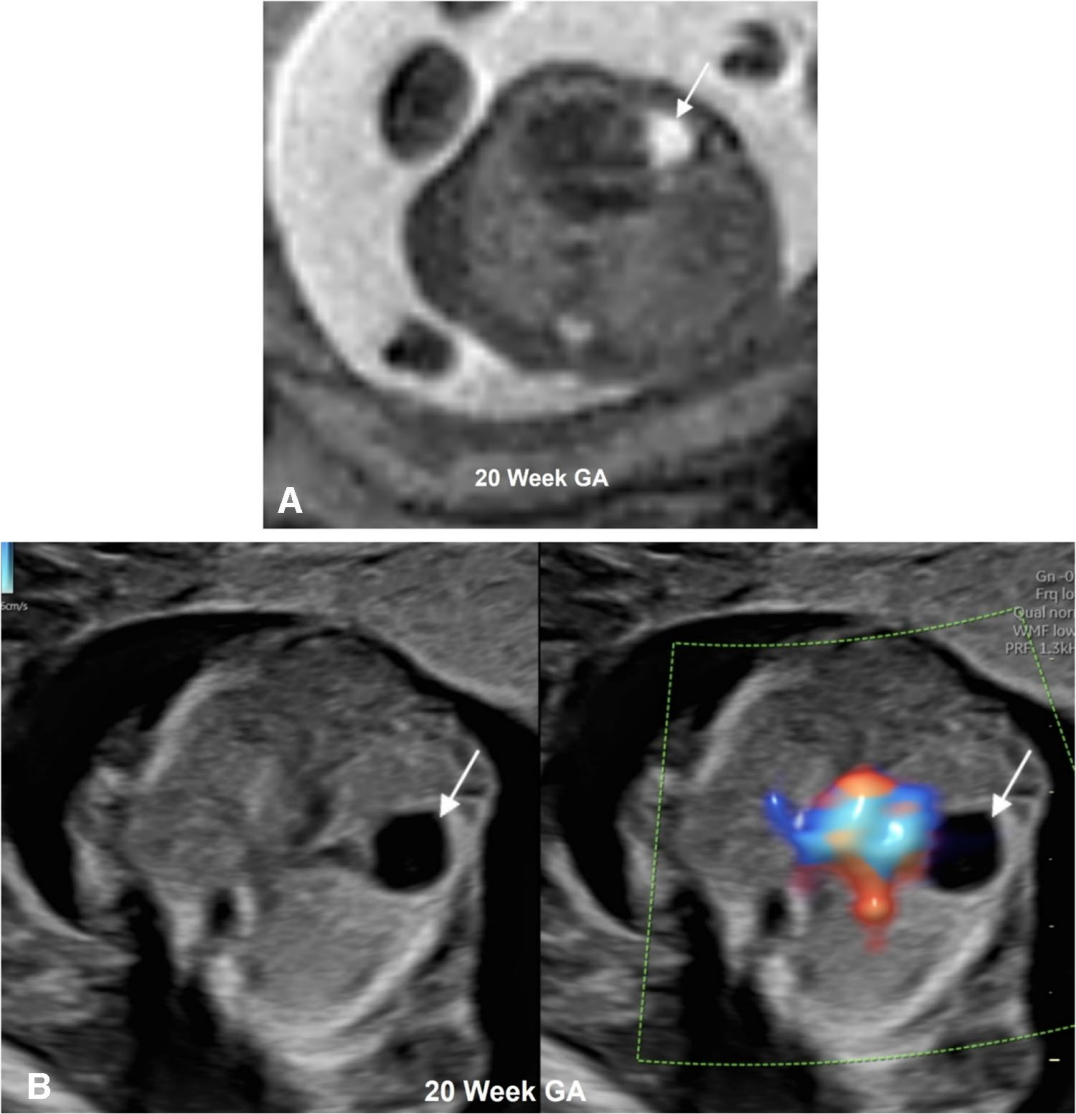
A Axial T2-weighted image in a 20-week gestation pregnancy showing a well-defined T2 hyperintense lesion (*arrow*) along the right cardiac border. B An axial fetal echocardiogram image in split screen view centered near the entrance of the inferior vena cava to the right atrium showing a well-defined anechoic cystic lesion (*arrow*) with no vascularity on color Doppler along the right cardiac border. *GA*, gestational age
